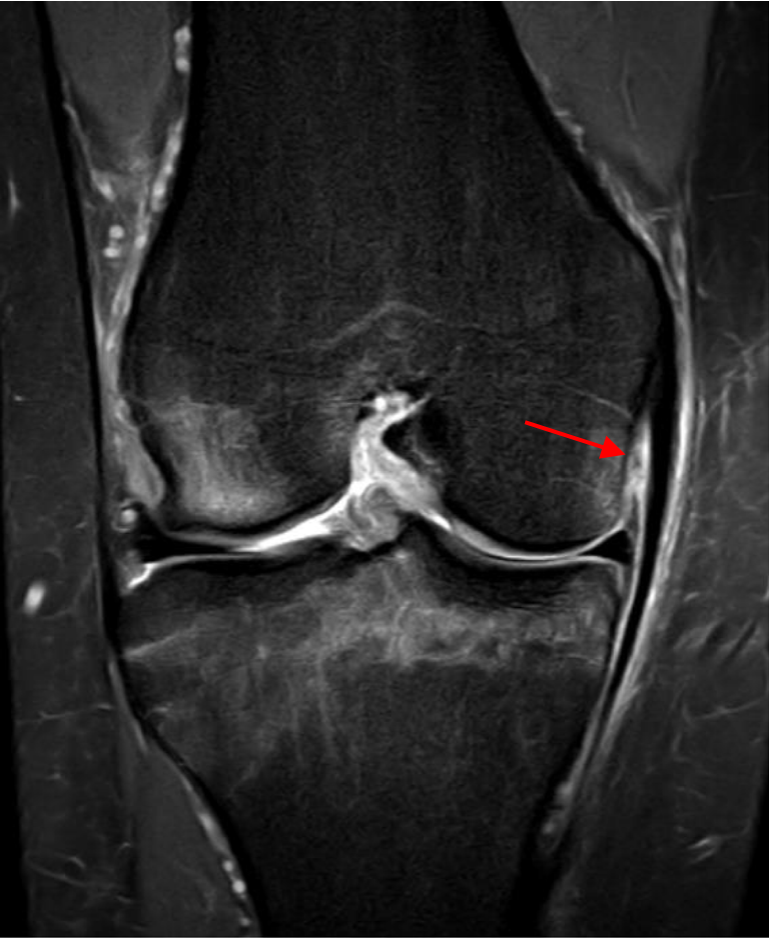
Isolated deep medial collateral ligament tear (meniscofemoral ligament) (arrow) on coronal proton density fat saturation magnetic resonance imaging sequence (right knee)
